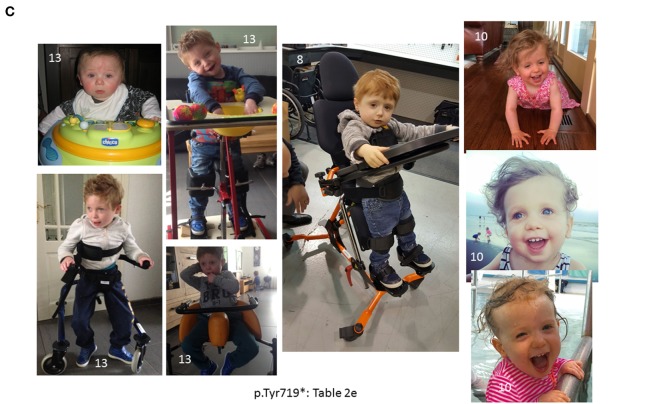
Pictures depicting AD’s development, brain development, and comparison to other children with the same mutation. (A) AD’s development in pictures: starting top left: young AD with her sister’s “push” to walk. AD is barefoot and her legs turned red from a very short walk. Her muscles in her ankles got tight and she exhibited low muscle tone and needed to be stretched several times a day, or she would suffer excruciating pain at night. The painful day walk and nights were full of crying periods. At the same time, a picture was taken, when she finally could lean against things with her leg braces and at a similar time point. Third picture on the top, AD at her first beauty pageant, after months of her sister and mother teaching her how to spin and dance with a baton ribbon. Fourth picture on the bottom, AD at her eighth birthday, just before her diagnosis and submission to brain scans. AD is successful at attending regular primary schools. Her smile keeps the family and caregivers going. (B) Magnetic resonance imaging results (volumetric T2 performed at 2007 and 2015). (C) Pictures of additional children with ADNP p.Tyr719* mutation (featured also in Table 2 (E), all materials given with parental informed consent).
